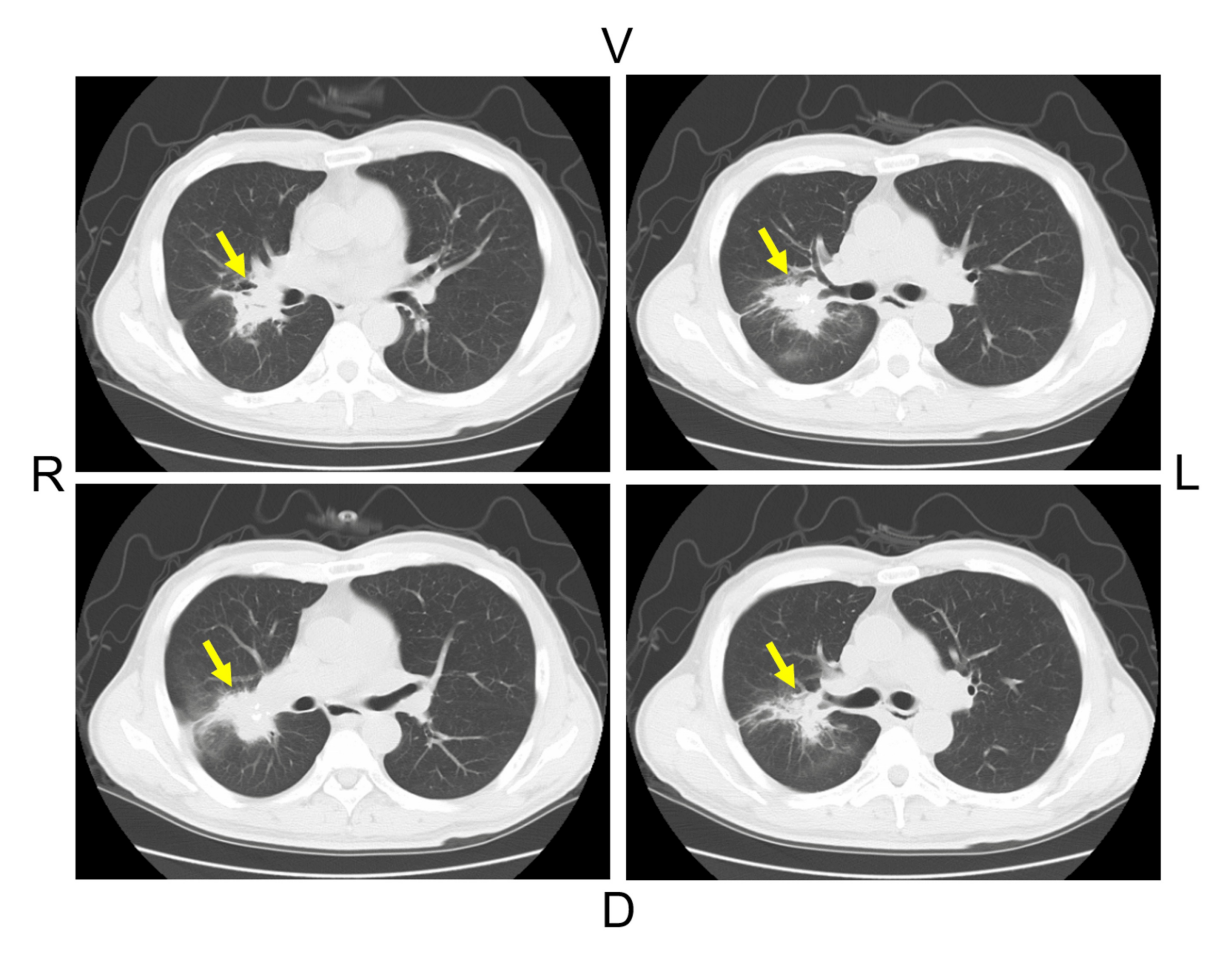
Contrast-enhanced computed tomography (CT) of the chest A nodular shadow with pleural traction and pleural retraction was observed in the right hilar region. R: right, L: left, V: ventral, D: dorsal.
